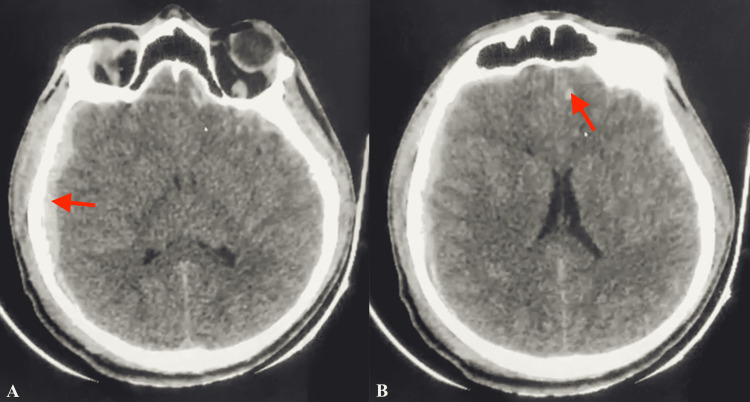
Preoperative axial non-injected CT scan showing the subdural hematoma, brain edema, and frontal contusions. A: Red arrow showing the fronto-temporal-occipital subdural hematoma. B: Red arrow showing the frontal contusion and brain edema. CT: computed tomography
